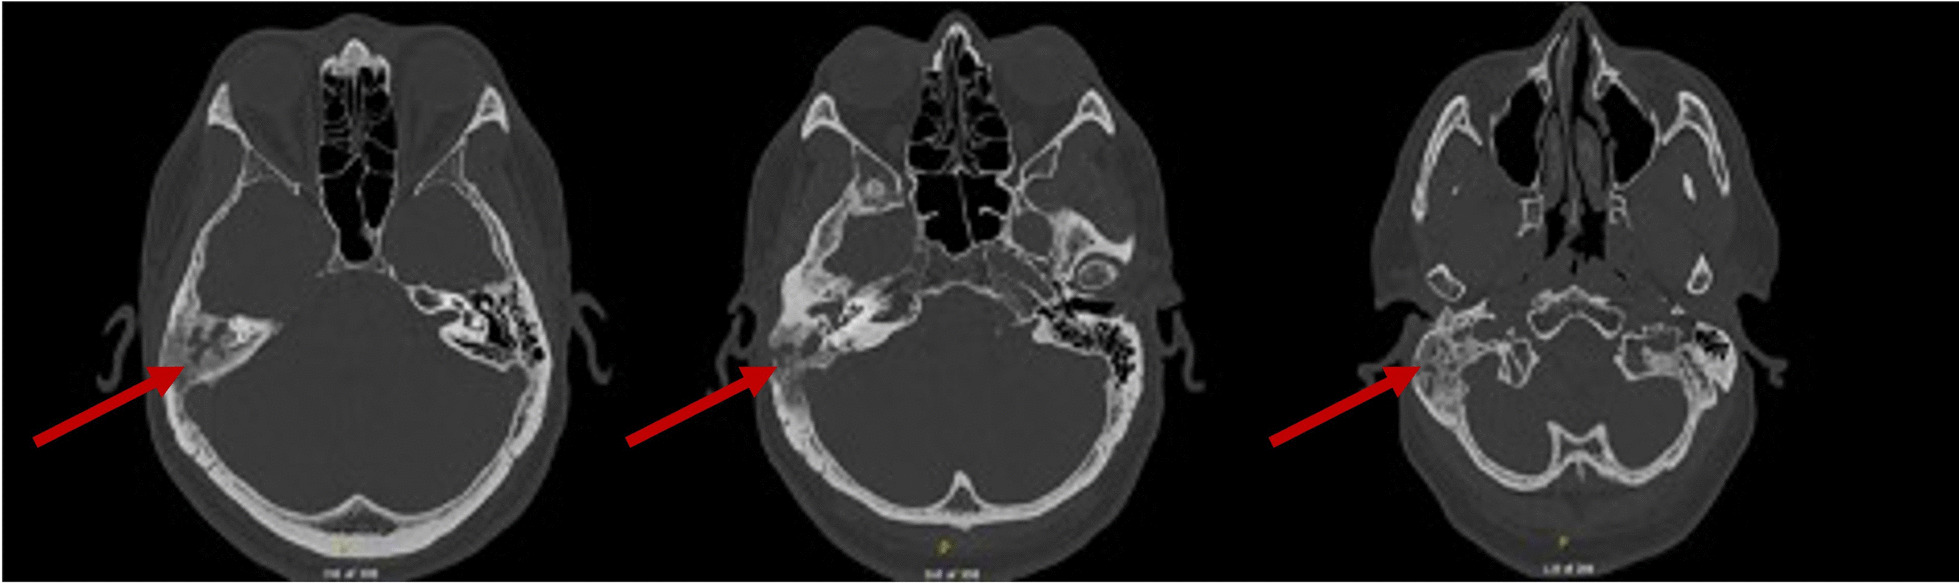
Three axial images of computed tomography temporal bone with arrow showing a lytic lesion in the right mastoid cavity
